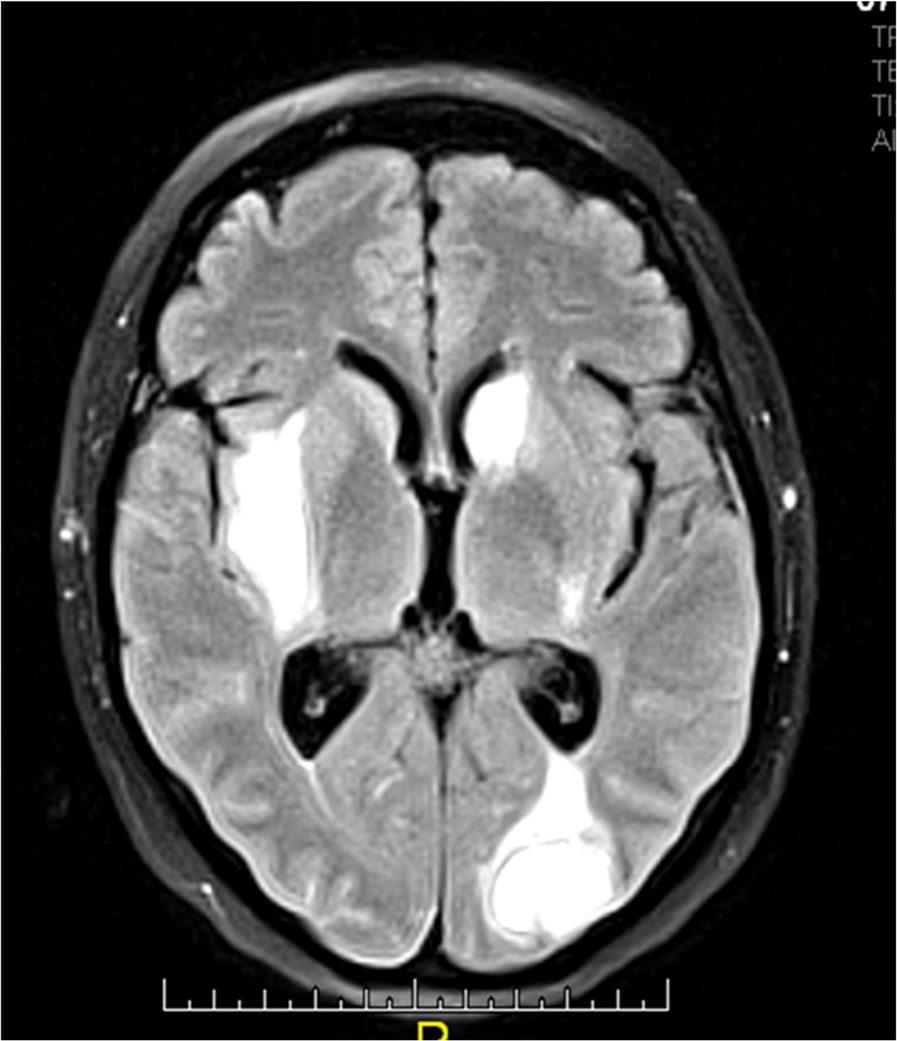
MRI brain showed multiple cerebral infarctions with hemorrhagic transformation.
